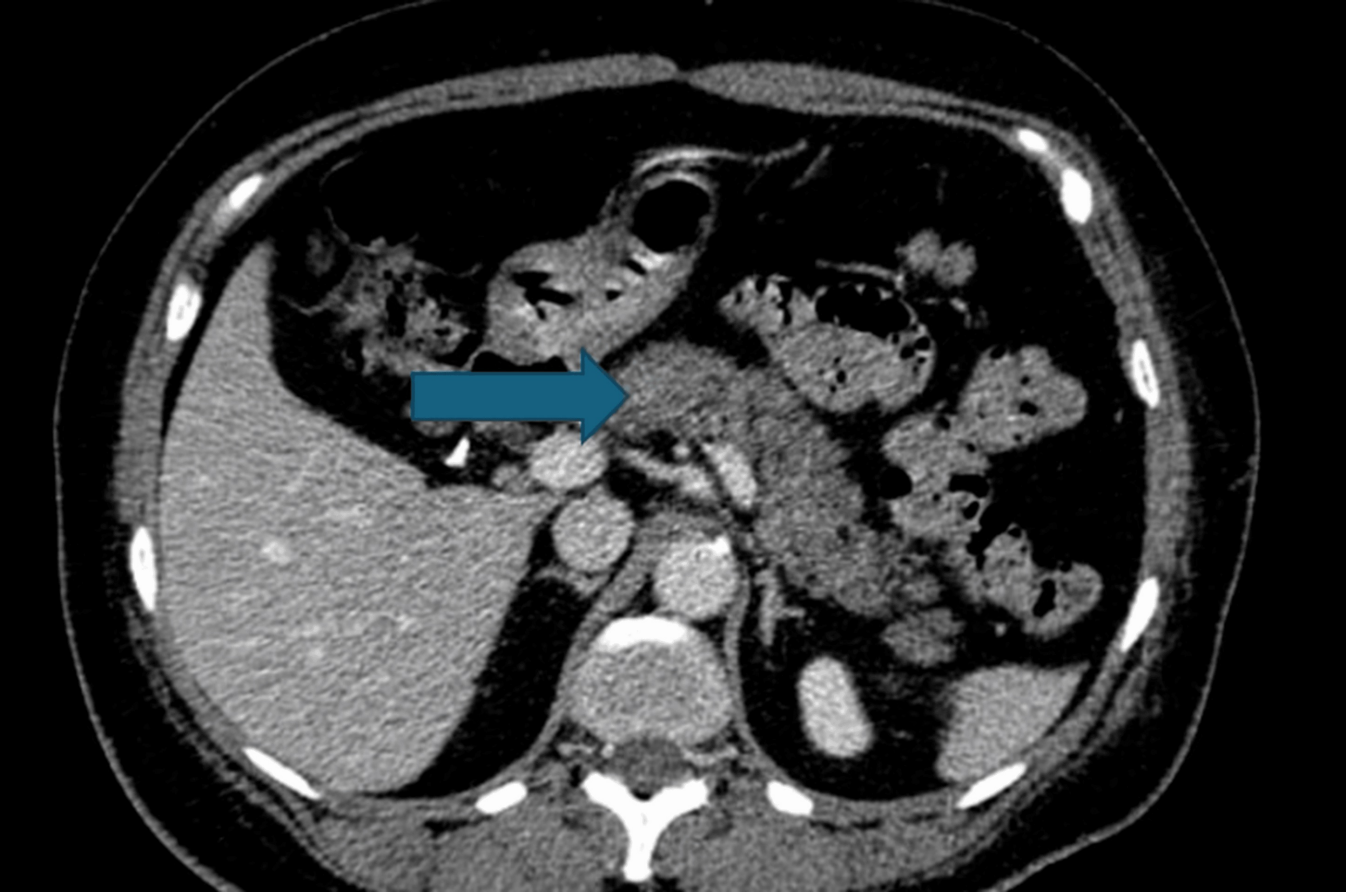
Acute pancreatitis on computed tomography An axial, contrast-enhanced computed tomography (CT) scan of the abdomen demonstrates inflammatory changes in the upper abdomen, characterized by peripancreatic fat stranding and soft tissue haziness concentrated around the head of the pancreas (indicated by the arrow). These findings are consistent with a diagnosis of acute interstitial pancreatitis.
